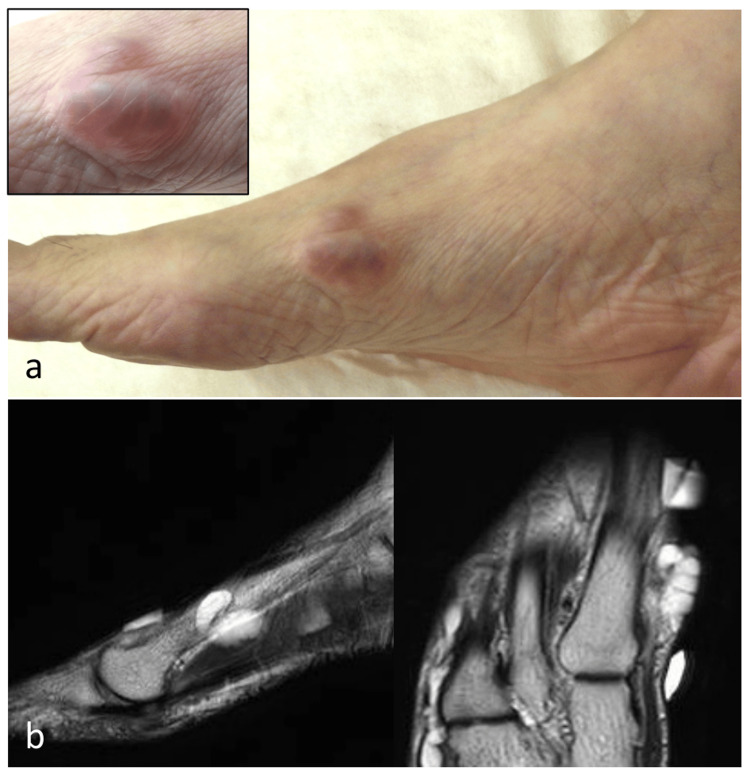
Clinical photograph and MRI findings a. A subcutaneous mass with elastic hardness and mobility over the underlying tissue is observed on the medial aspect of the first metatarsal. The lesion is visible beneath the skin and was associated with mild tenderness. b. Preoperative T2-weighted MRI shows a multilocular cystic lesion with high signal intensity; however, clear continuity with the first metatarsophalangeal joint was not identified.
